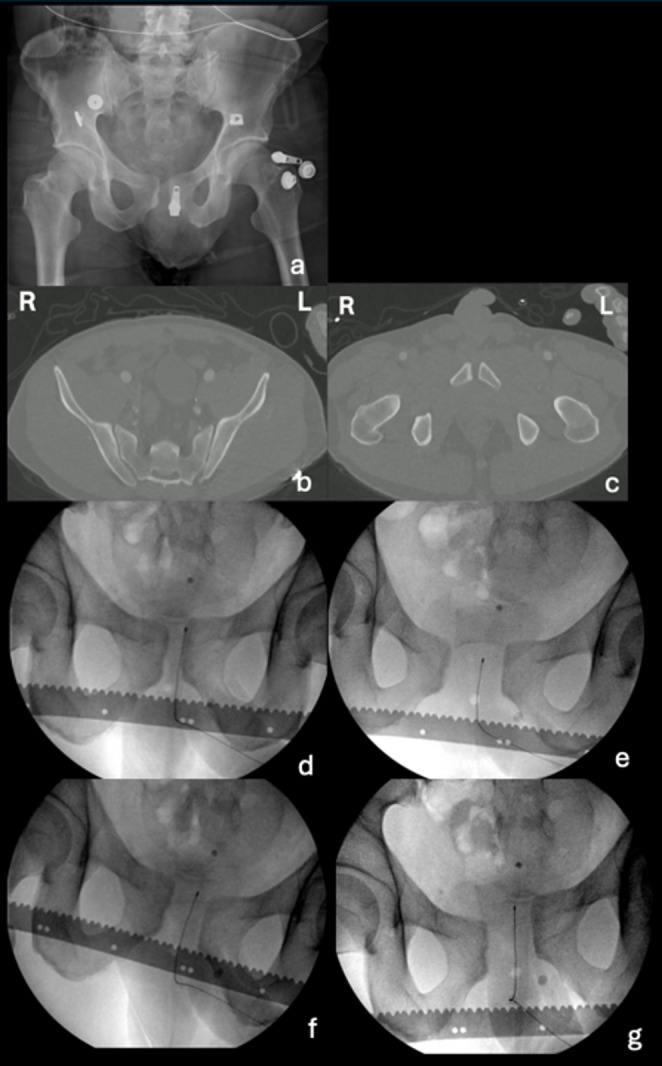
Case 5, a AP static radiograph (artifacts on image are pieces of metal from clothing), b CT scan showing the sacroiliac joint, c CT scan showing pubic symphysis, d AP EUA image in endorotation stress (lateral compression), e AP EUA image in exorotation stress (frog position), f AP EUA image in push right leg/ pull left leg stress (rotational instability), g AP EUA image in push left leg/ pull right leg stress (rotational instability)
